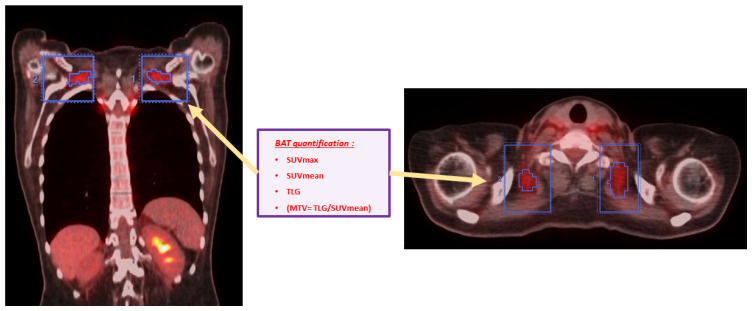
Delineation of BAT volume and measurement of the quantitative PET parameters in ^18^F-FDG PET/CT scans in our patients.
